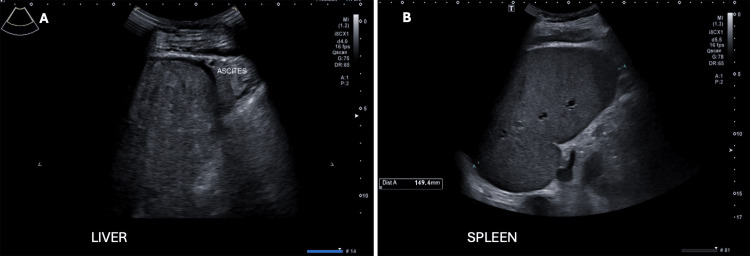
Pre-operative abdominal ultrasound with liver demonstrating a coarsened echotexture and nodular surface contour with small volume ascites, consistent with cirrhosis (A), and splenomegaly (B)
